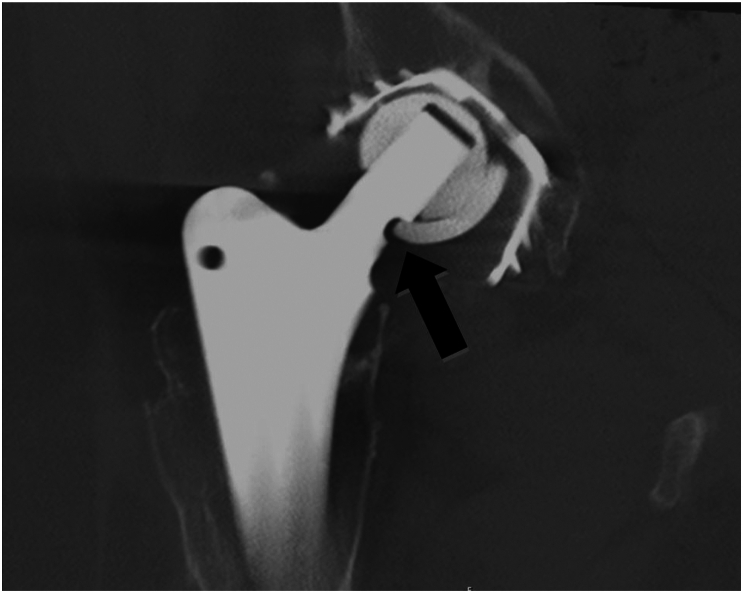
Computed tomography of the right hip, CT scan showing the Femoral Neck-Bite Sign (black arrow) due to a ceramic liner fracture, note the large osteolytic areas extending from the cup into the pelvis and surrounding the stem. Also of interest is a thinning of the acetabular cup in its superior aspect presumably caused by abrasive wear. The cup inclination as measured in the CT scan is 47 degrees and cup anteversion is 22 degrees. CT, computed tomography.
